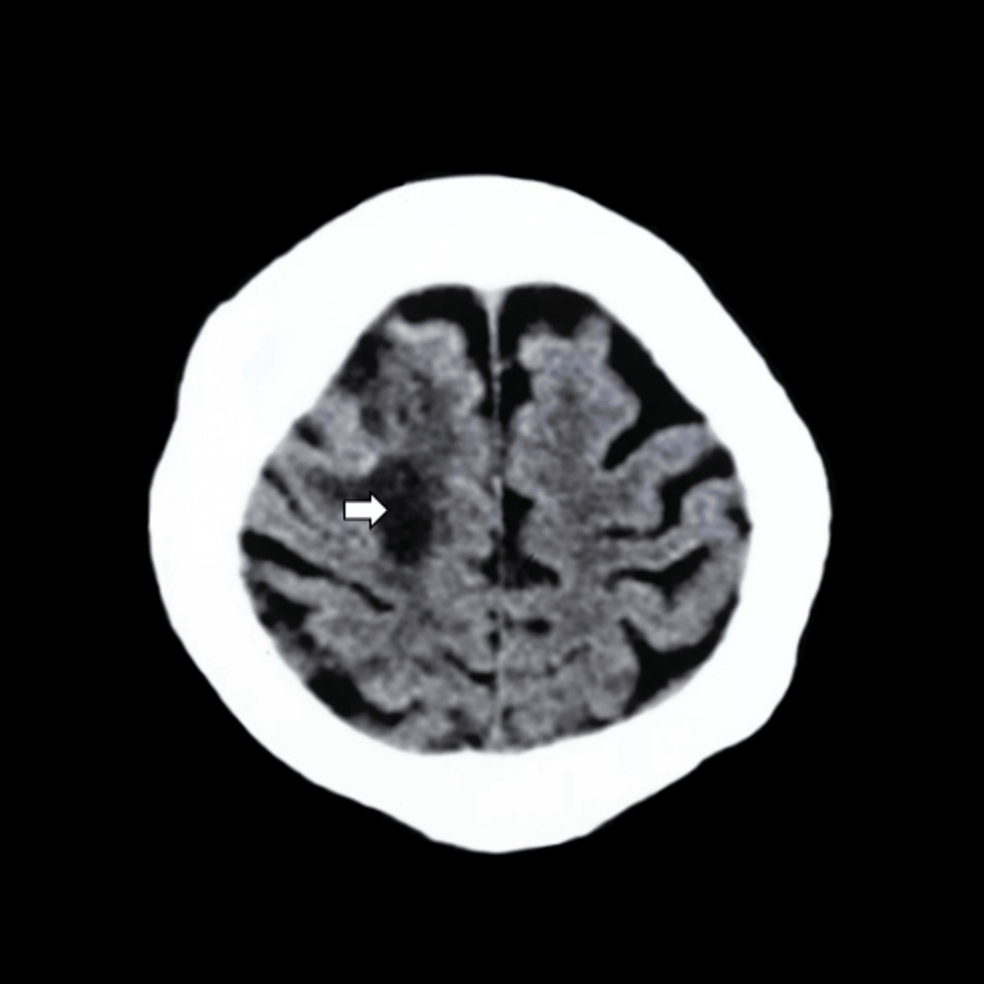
An axial CT scan of the head two months after therapy initiation showing a hypodense sequelae lesion (white arrow) with a marked reduction of the perilesional edema. CT: computed tomography
